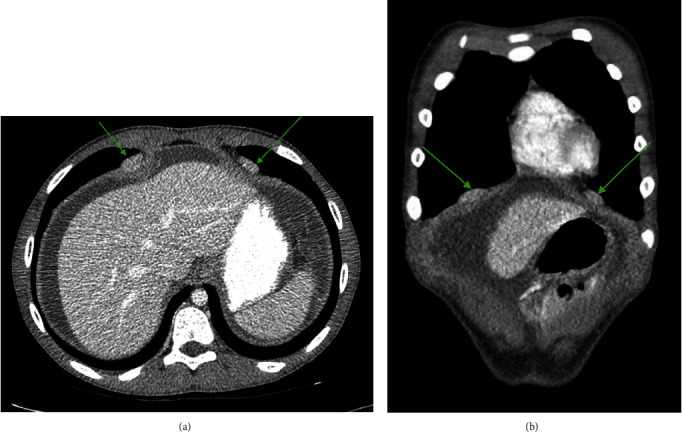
(a) Axial CT with intravenous contrast of the chest demonstrating pleural-based nodules. (b) Coronal CT with intravenous contrast of the chest demonstrating pleural nodules.
